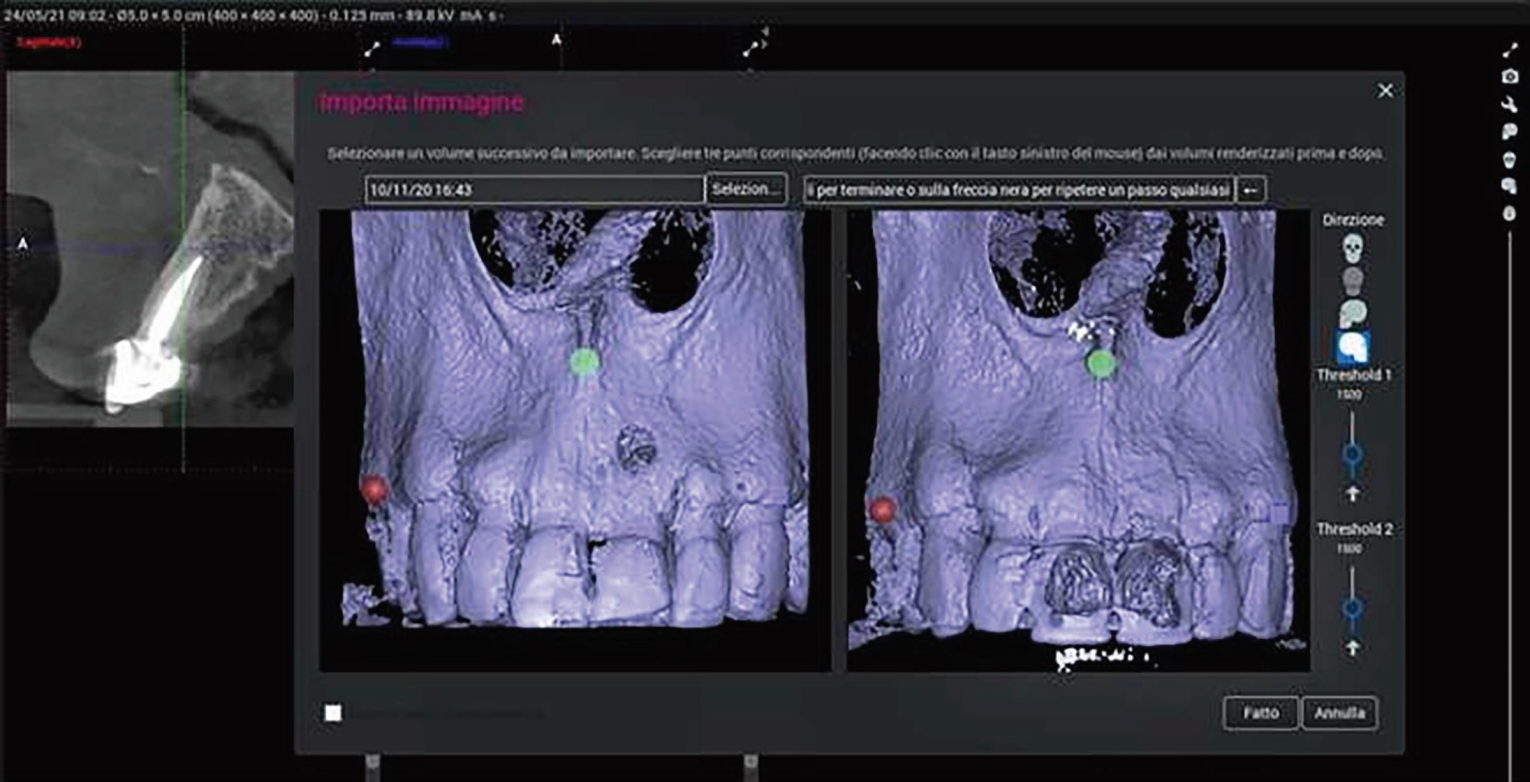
Alignment of preoperative (T0) and follow-up (T1) CBCT scans using stable anatomical landmarks (palatal vault, anterior nasal spine, and adjacent teeth) for superimposition.
